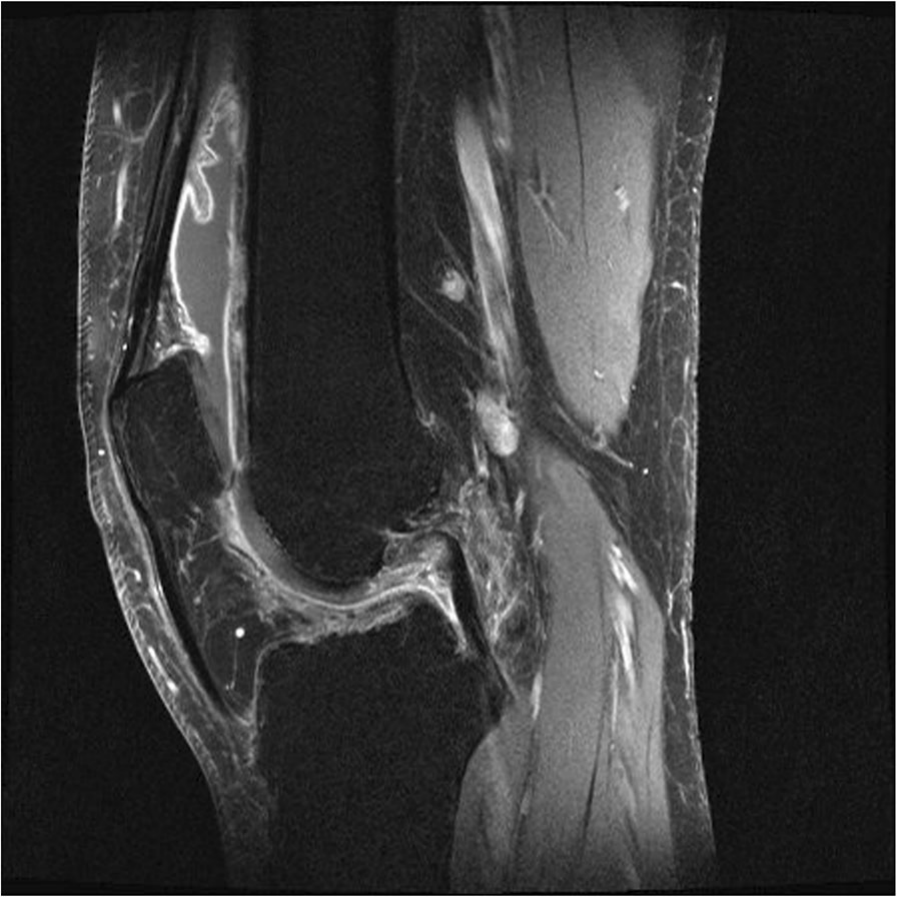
Saggital T2 MRI of the knee with fat saturation showing extensive intra-articular inflamed synovia and a ruptured ACL
